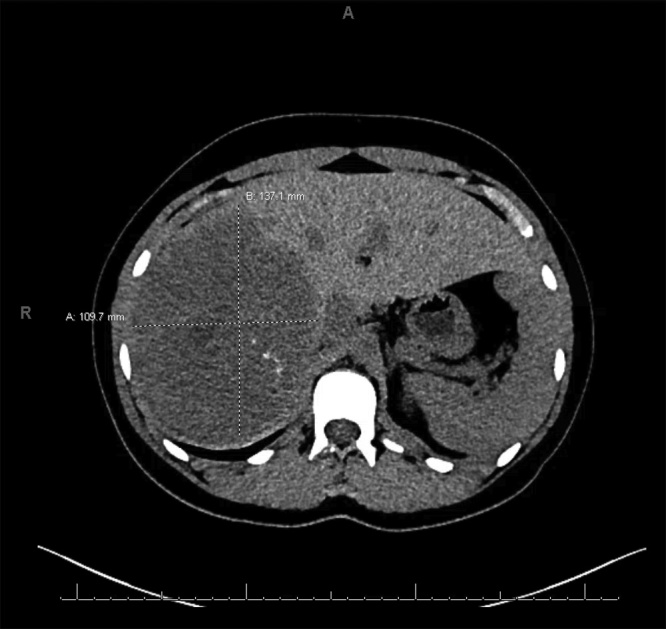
Triphasic abdominal CT scan of patient’s abdomen with CNSET indicated.
